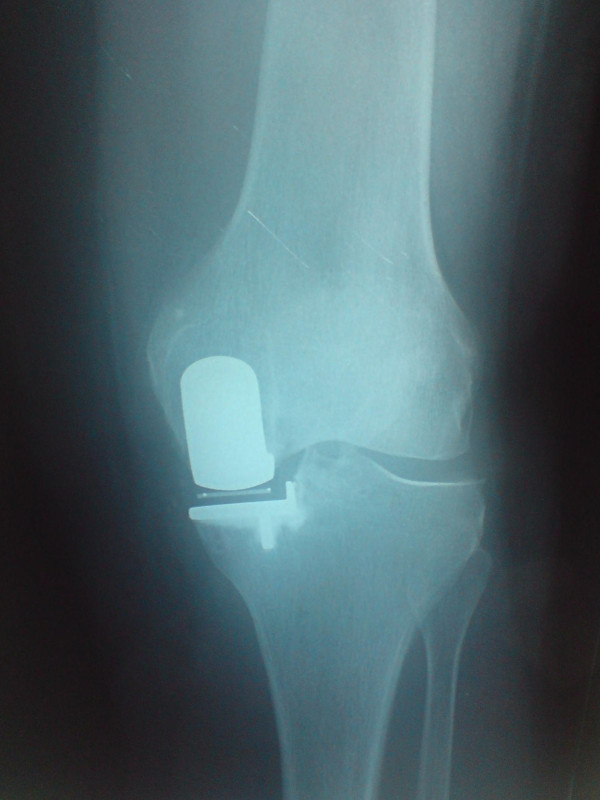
An example of a knee with a good radiographic score.
